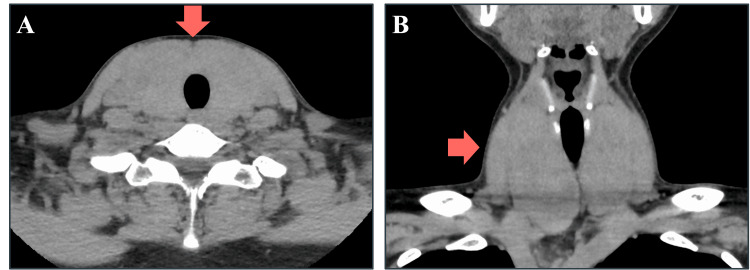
CT image of the thyroid The thyroid gland was enlarged to a maximum width of approximately 7 cm. (A) Axial CT scan showing a diffusely enlarged thyroid gland. (B) Coronal CT scan displaying the same diffuse enlargement of the thyroid gland.
